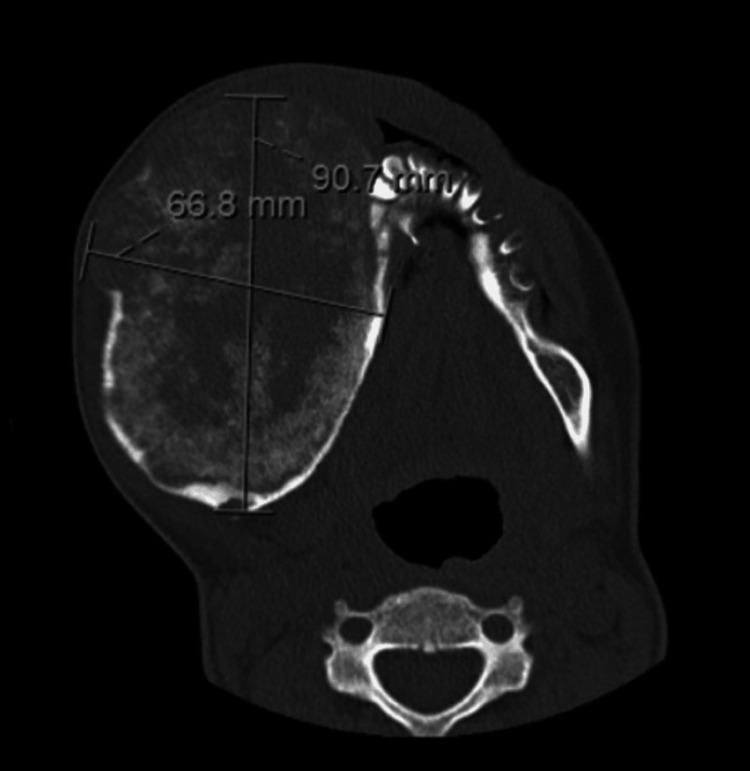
Axial CT showing the right mandibular mass measures 6.7 x 9.1 x 7.6 cm.
